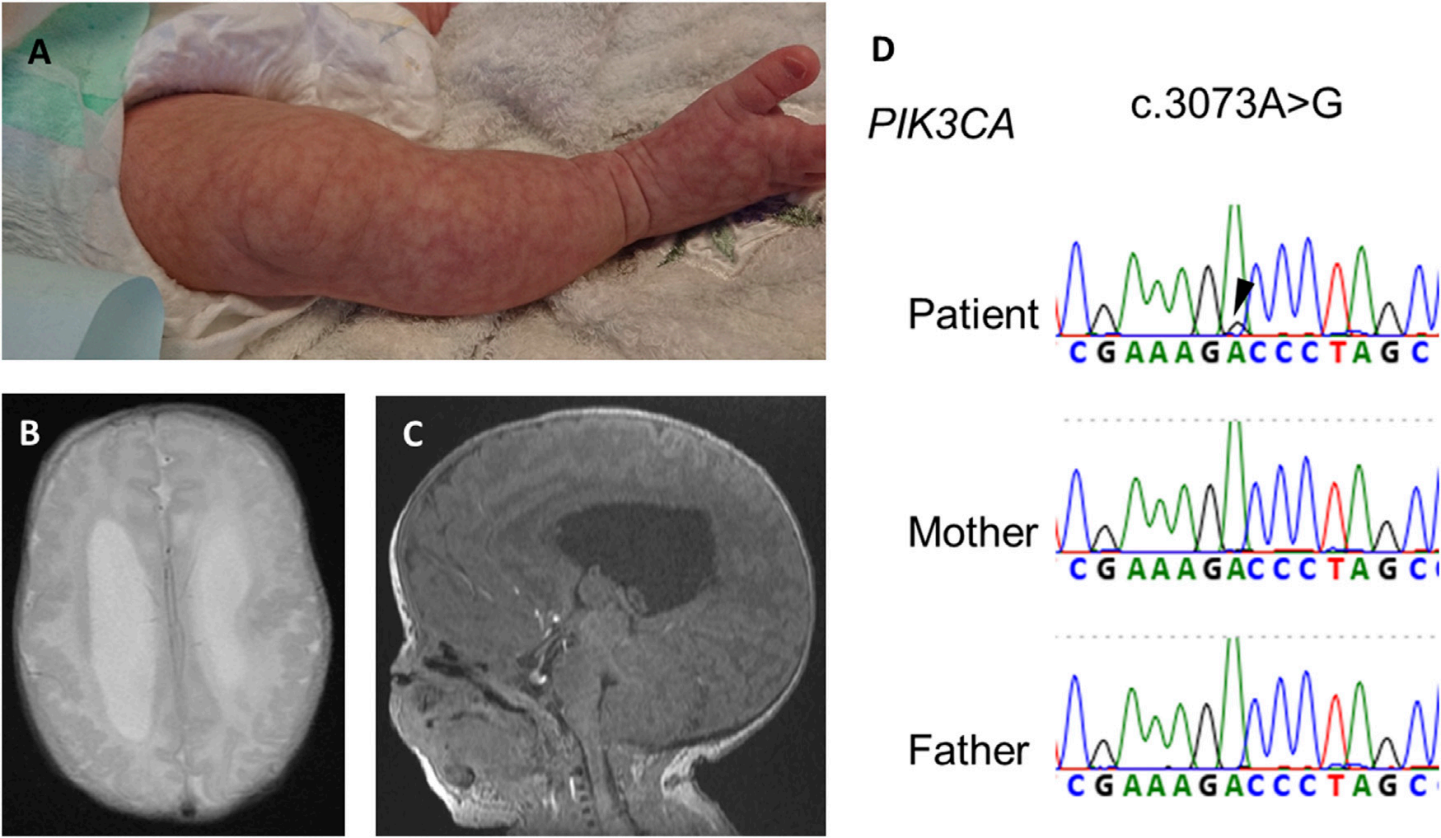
Physical and neuroimaging findings at the neonatal period. Cutis marmorata and sandal gap toe in the right leg (A), extensive bilateral polymicrogyria and ventricular dilatation [(B), axial t2-weighted image], and mild sagging of the central cerebellum [(C), sagittal T1-weighted image]. (D) Electropherogram of Sanger sequencing of the patient and his parents. Black arrowheads indicates a somatic mosaic *PIK3CA* variant.
